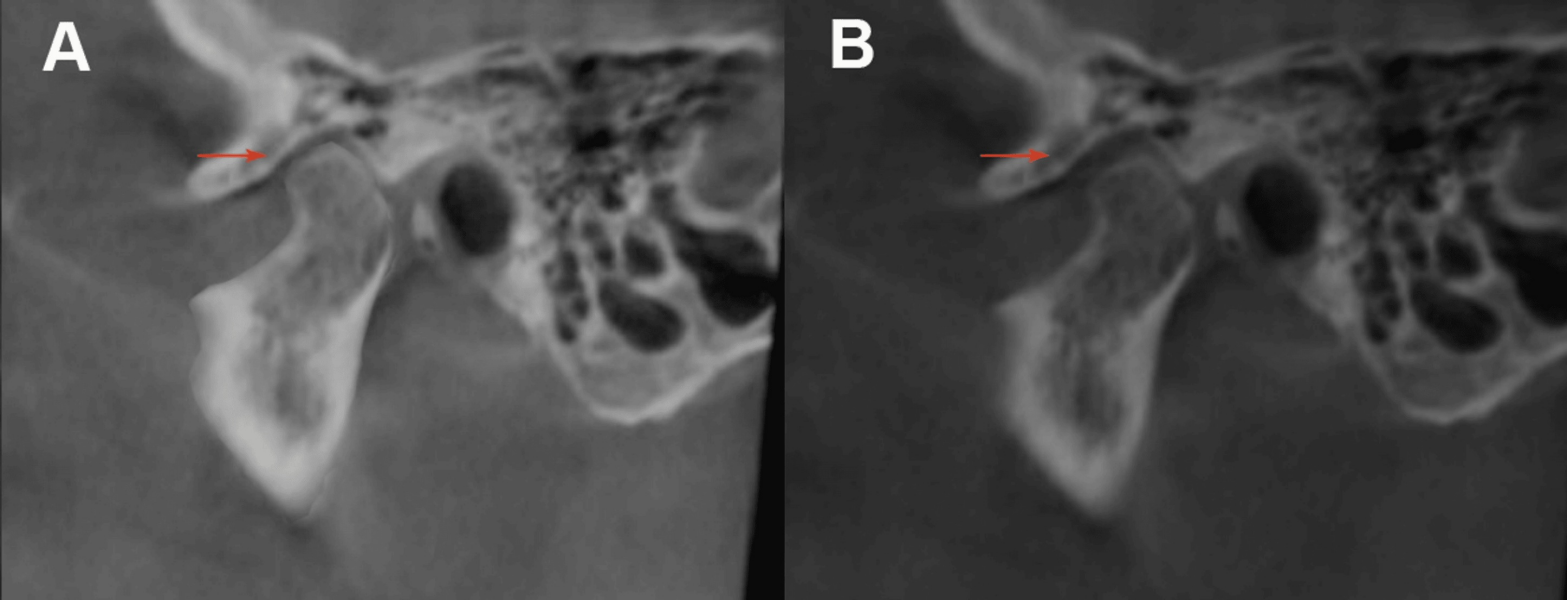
CBCT of patient showing difference in anterior space of TMJ in sagittal plane: (A) time of denture insertion; (B) after one-year interval. CBCT: cone-beam computed tomography, TMJ: temporomandibular joint.
